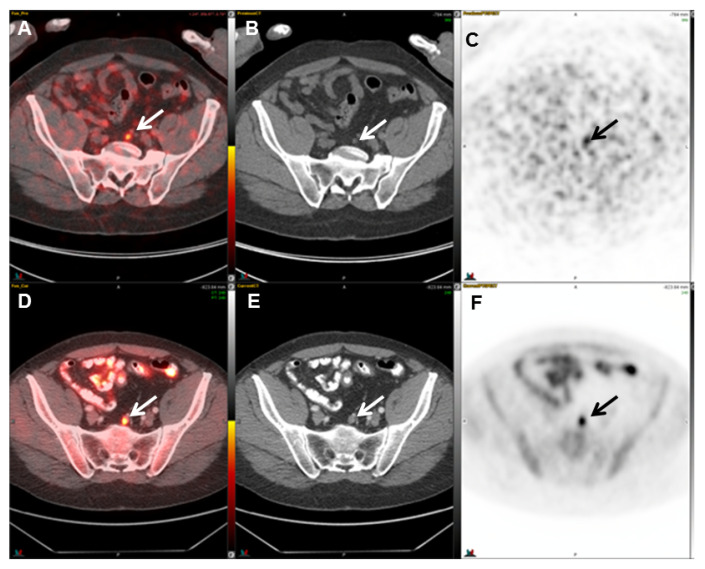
A 74-year-old man with prostate adenocarcinoma status post radical prostatectomy and biochemical recurrence. Axial fused PET/CT (A), CT (B), and PET (C) images demonstrate focal increased ^68^Ga-PSMA-11 activity (SUVmax = 4.2) localizing to a 0.7 cm lymph node in the pelvis (arrows), consistent with nodal metastasis. Axial fused PET/CT (D), CT (E), and PET (F) images of an ^11^C-choline study obtained 4 months later demonstrate focal increased ^11^C-choline activity (SUVmax = 4.8) localizing to the same lymph node, which has enlarged, measuring 1.0 cm (arrows).
